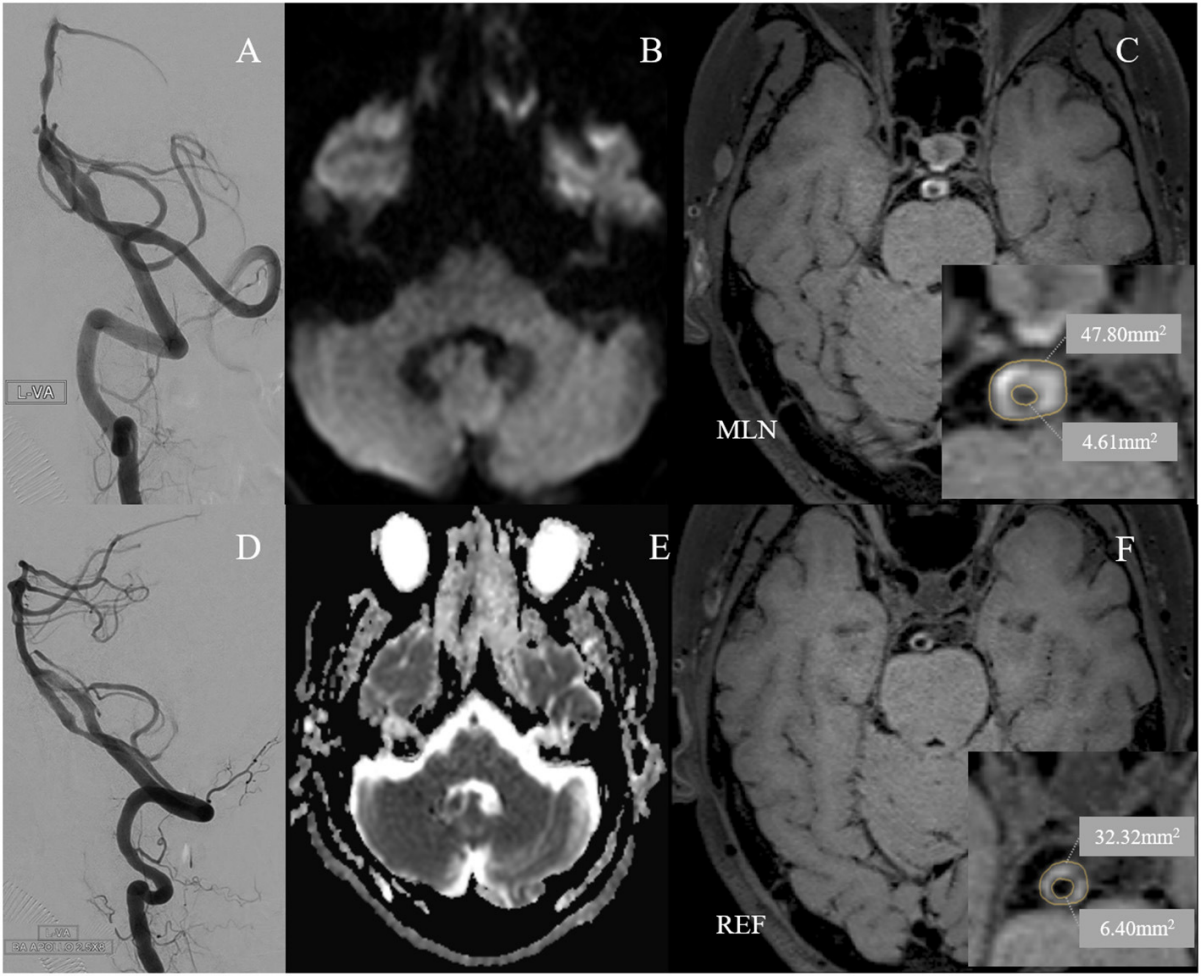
A 60-year-old man with cigarette smoking history presented with recurrent transient attacks of vertigo for 1 month. (A) Pre-operative digital subtraction angiography (DSA) showed stenosis at the low segment of the basilar artery (BA) with 77.7% of degree. (D) A 2.5 × 8 mm Apollo balloon-mounted stent (MicroPort NeuroTech, Shanghai, China) was placed at the stenotic segment. There was no new cerebral infarction confirmed by diffusion-weighted imaging (B) and apparent diffusion coefficient imaging (E) within 72 h after stenting. Figures of the right column are cross-sectional T1-weighted BA images at the maximal lumen narrowing (MLN) (C) and reference (REF) (F) sites. The plaque was concentric and belonged to the circular type (C). Vessel area (VA) and lumen area (LA) at the MLN (C, VA 47.80 mm^2^, LA 4.61 mm^2^) or REF (F, VA 32.32 mm^2^, LA 6.40 mm^2^) sites were manually traced for measuring after zooming in 400 times. Wall area (WA) at the MLN or REF sites was calculated by VA-LA. The plaque burden was calculated as [(WA _MLN_-WA _REF_)/VA _MLN_] × 100% and the remodeling index was calculated as VA _MLN_/VA _REF_. Therefore, the plaque burden was 36.1%. The remodeling index of the vessel at MLN was 1.48, which was categorized as positive remodeling.
